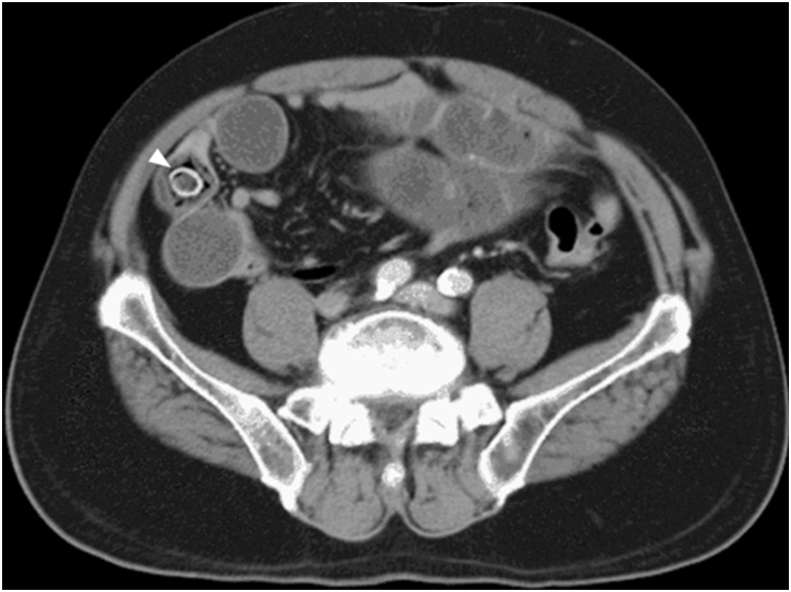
Abdomen computed tomography axial view demonstrating small bowel obstruction with a transition point in the right lower quadrant, associated with mottled intraluminal gas and a rim-calcified intraluminal lesion (arrowhead) proximal to the transition zone.
